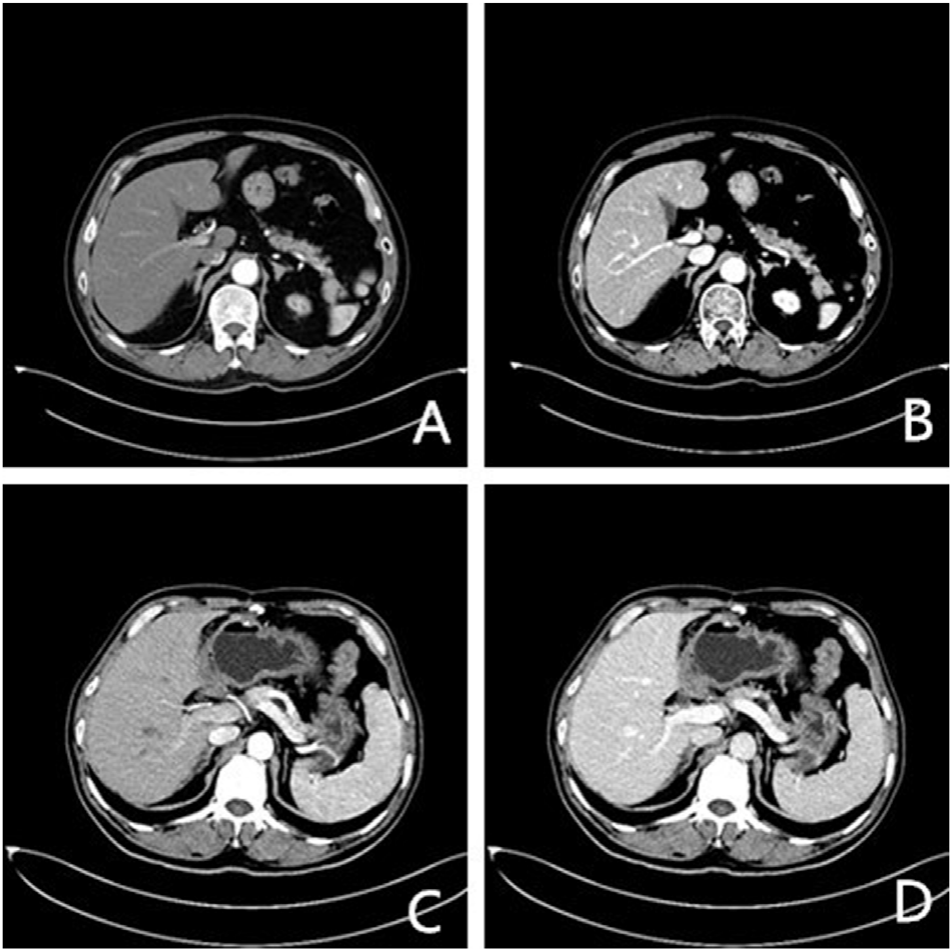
Axial enhanced CT images of two patients (arterial and venous phase). (A), (B) a 56-year-old man with lung cancer after chemotherapy (400 mg I/ml); (C), (D) a 64-year-old man after resection of gastric cancer (300 mg I/ml).
